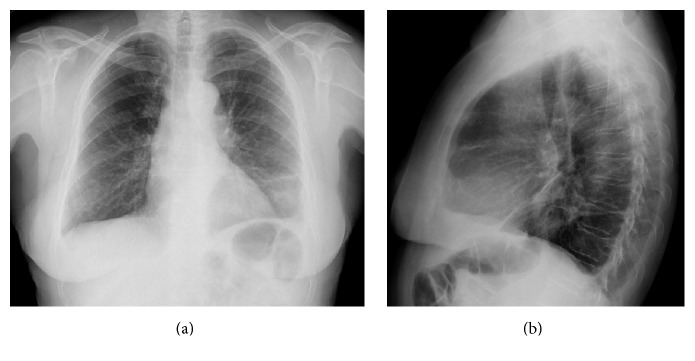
Posteroanterior (a) and lateral (b) chest X-rays. The chest radiography showed a peripheral lesion in the left lower lung and a small volume of pleural effusion on the same side.
